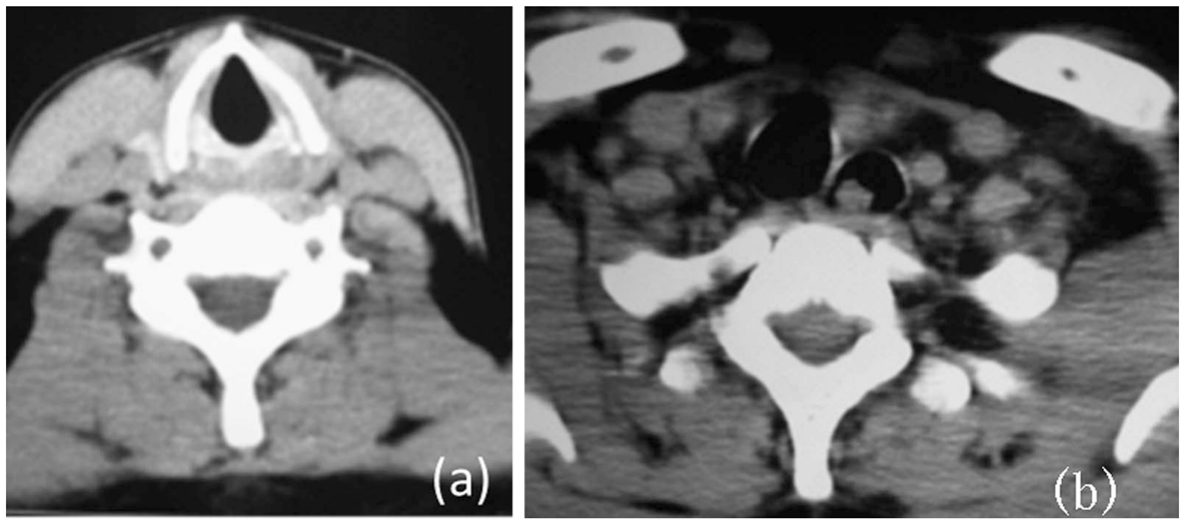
CT showing the mass in the esophagus. (a) The larynx was normal. (b) The esophagus was dilated and the left bronchus was compressed (arrow shows the mass).
